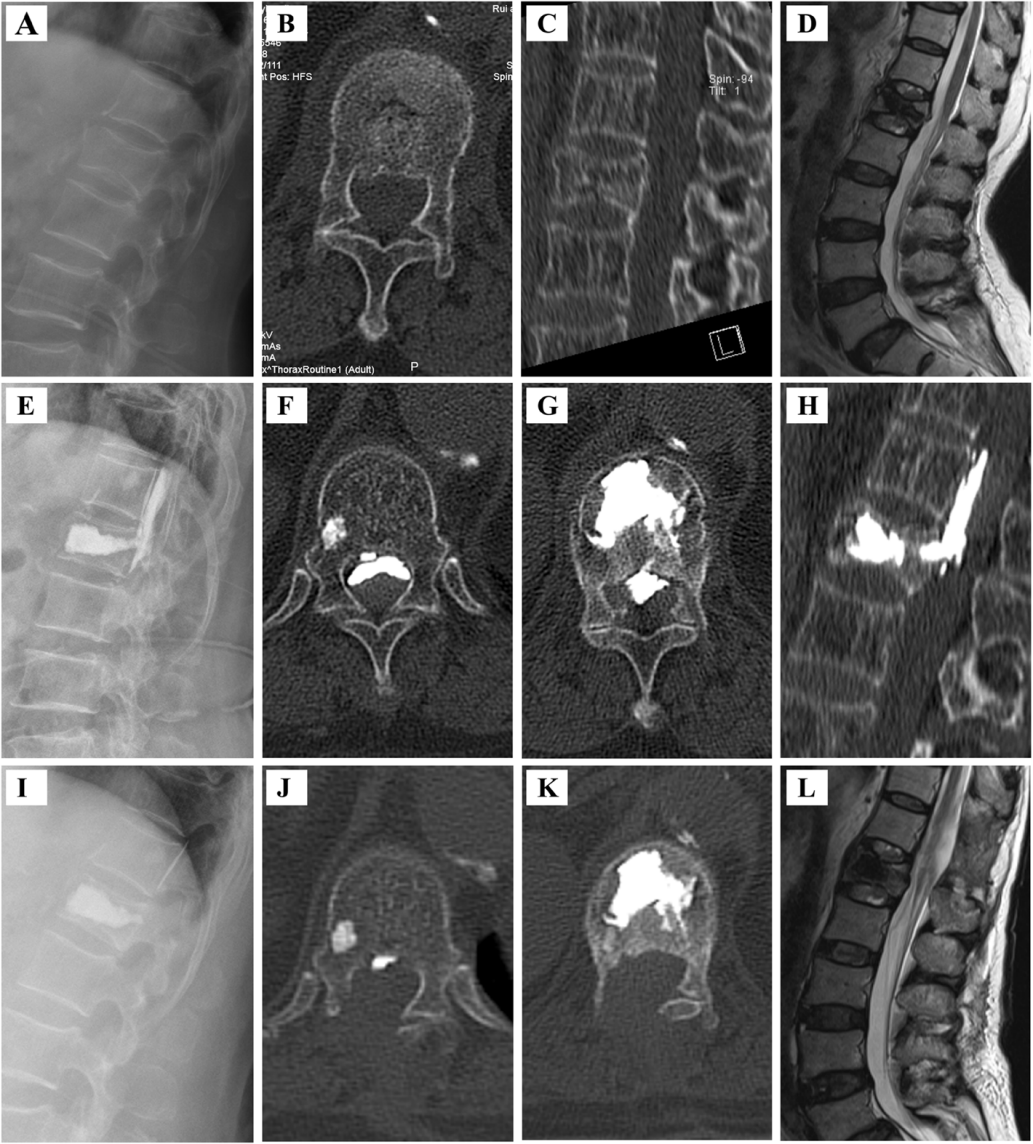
PKP surgical procedure for the treatment of a 69-year-old female patient with OVBFs in L_1_ vertebra. A Preoperative lateral radiograph showing a burst fracture of L_1_. B, C Preoperative CT-scan (plain and sagittal reconstruction image) showing the burst fracture with spinal canal compromise. D Preoperative MRI (T2-weighted sequences) showing the burst fracture with spinal canal compromise. E Postoperative lateral radiograph showing cement leaking from T_12_ to L_1_. F, G, H Postoperative CT-scan (plain at T_12_ and L_1,_ and sagittal reconstruction image) showing cement leaking with spinal canal compromise. I Lateral radiograph showing removed cement leaking. J, K CT-scan (plain at T_12_ and L_1_) showing removed cement leaking. L MRI (T2-weighted sequences) 2 years after surgery showing reduced spinal canal compromise
